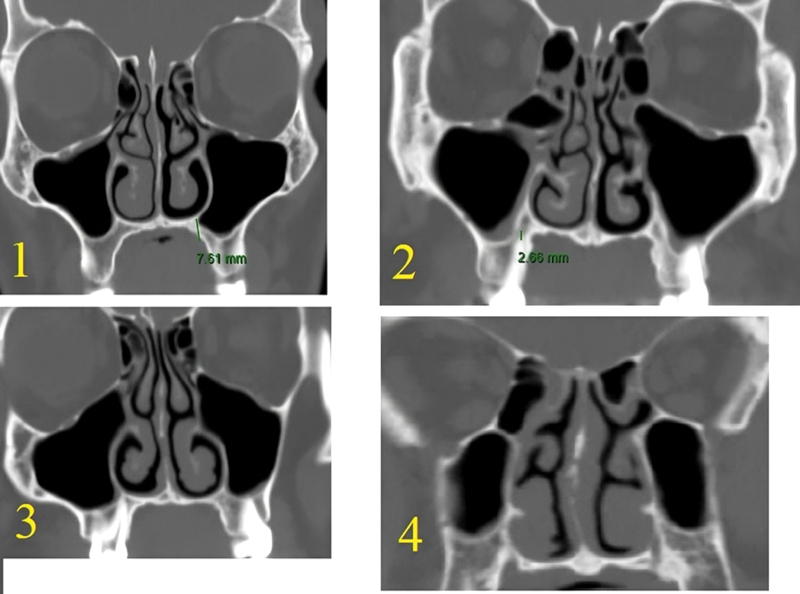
A CT scan of the different grades of the maxillary sinus floor.
